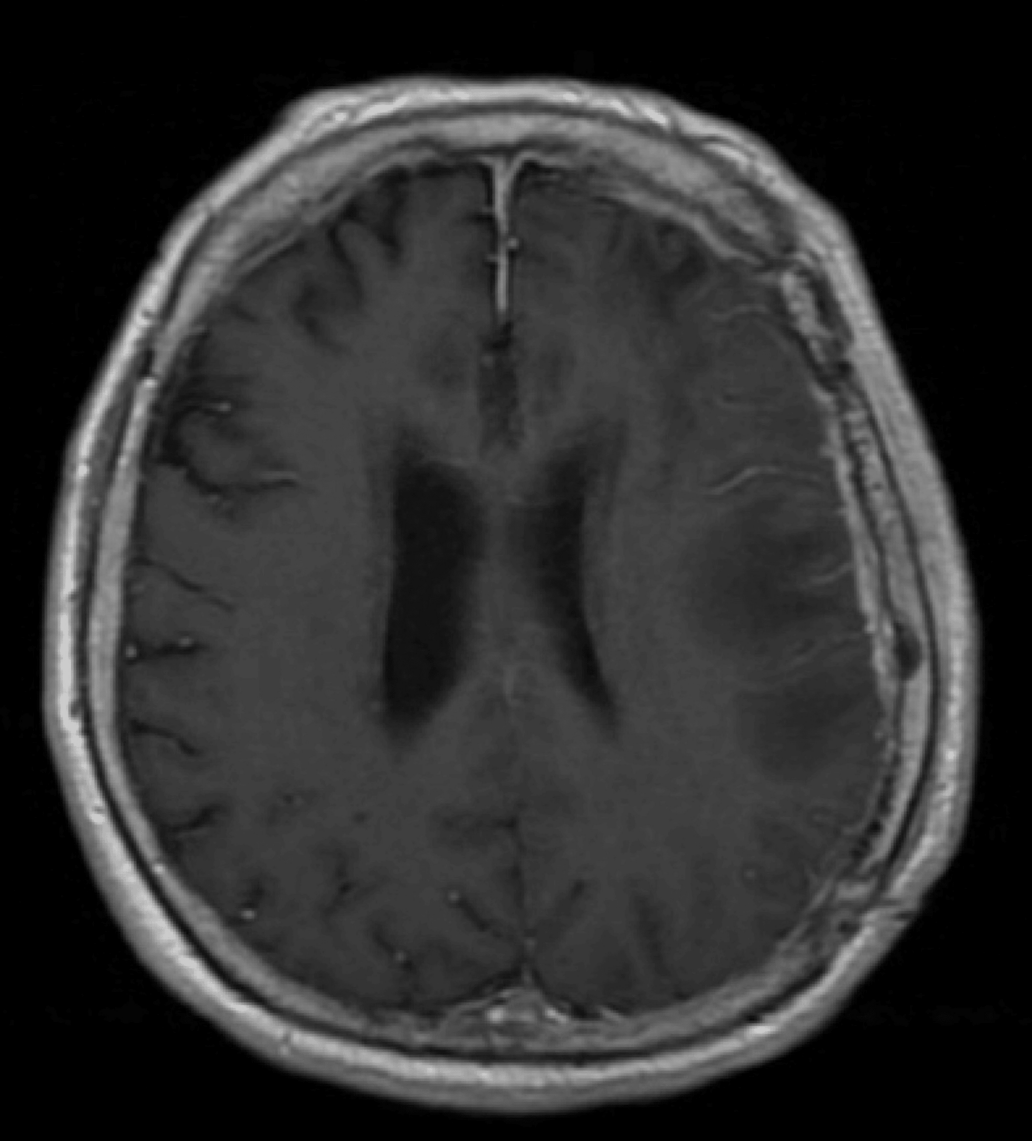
Initial Presentation MRI of the brain displays pachymeningeal enhancement with underlying parenchymal edema, prompting immediate surgery.
